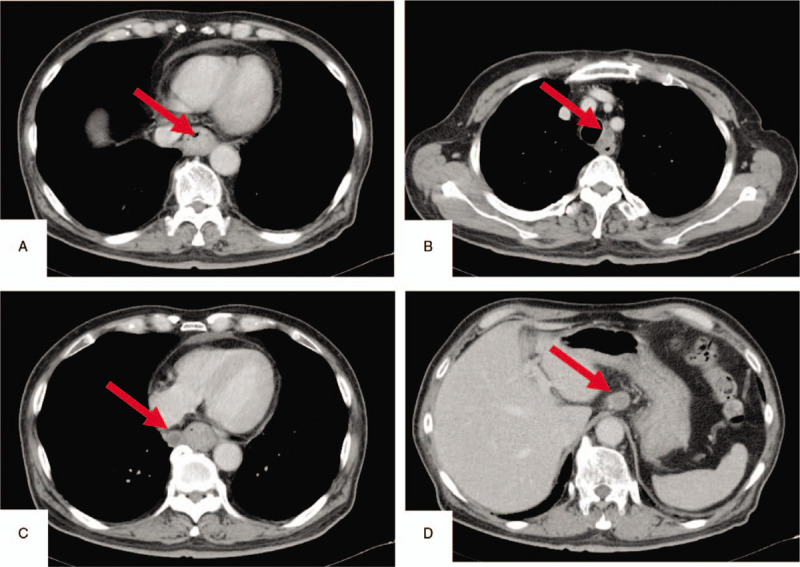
a-d Contrast-enhanced computed tomography showed the tumor occupying the lumen of the lower thoracic esophagus (a). Computed tomography also revealed enlarged lymph nodes, namely, the left upper paratracheal (b), lower paraesophageal (c), and lesser curvature (d) lymph nodes.
